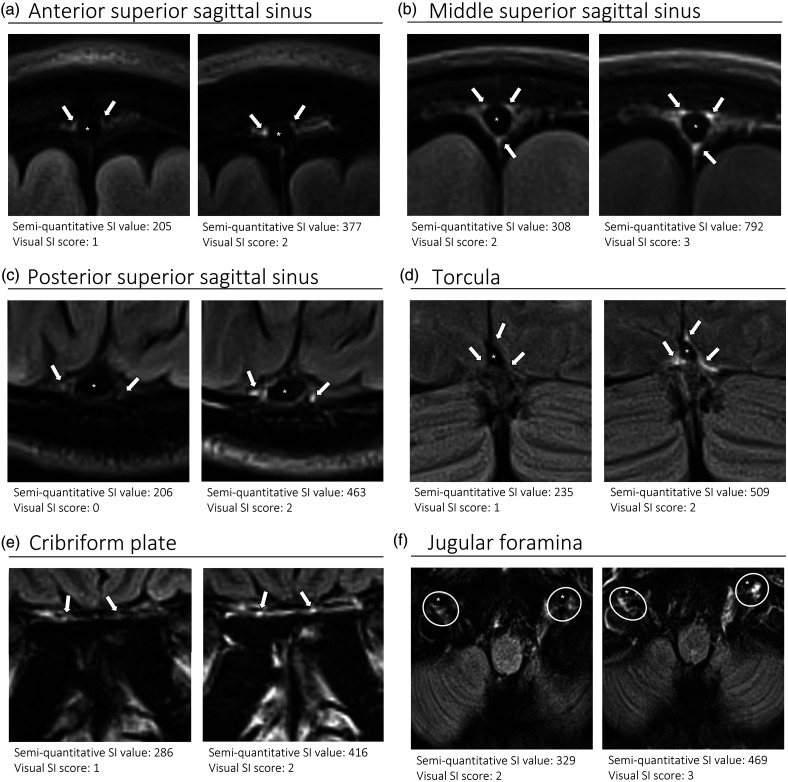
Representative T2 FLAIR images showing signal intensity in dorsal and basal structures. Pre- (left side of all panels) and post-contrast (right side of all panels) in the same subject for each area of assessment (panels a–f) (different subjects for different areas).The measured semi-quantitative SI values in arbitrary units (the average of all regions of interest measured at the specific area) and qualitative, visual SI scores are indicated below the images from the individual subjects. For the areas along the superior sagittal sinus (SSS) there were punctate hyperintensities at either side and inferiorly to the sinus, representing meningeal lymphatics in cross section. The hyperintensities at the cribriform plate and jugular foramina were more diffuse. For the latter, the white circles indicate the area of the pars nervosa and not individual regions of interest. The arrows and asterisks indicate meningeal lymphatic structures and the lumen of venous vessels (SSS, jugular vein), respectively. SI: signal intensity.
